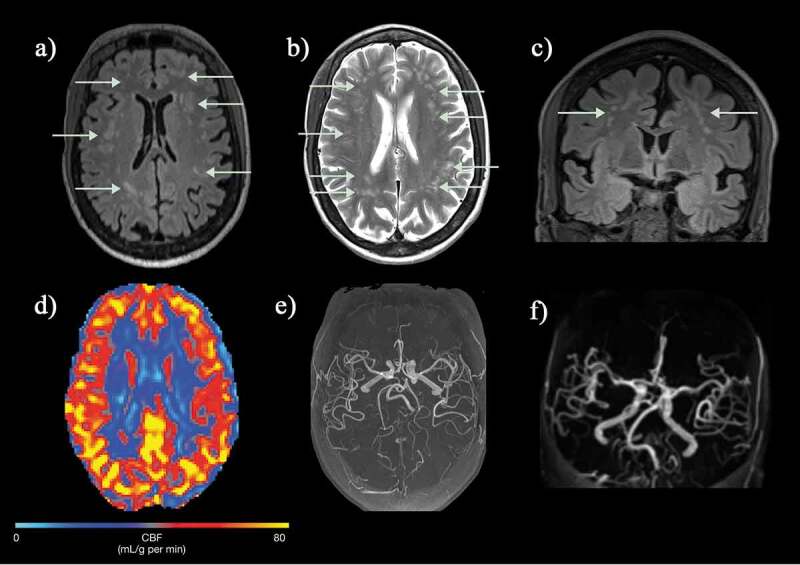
(a, b) Axial FLAIR and T2-weighted images and (c) coronal FLAIR images in a normally functioning 57-year-old female SCD patient with no neurological deficits who was scanned as part of a study and found to have widespread white matter lesions in the subcortical and deep white matter regions. (d) Cerebral blood flow map (CBF) map showed slightly reduced perfusion anteriorly and (e, f) intracranial MRA was entirely normal. FLAIR = fluid attenuated inversion recovery
